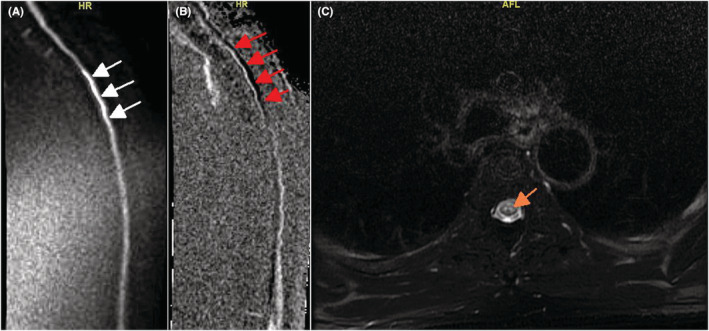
Sagittal plane of MRI spine DWI sequence (A, white arrows) and ADC (B, red arrows) showing restricted diffusion suggesting acute spinal cord infarction from T3 to T5 levels. Axial plane T2‐weighted sequence of MRI spine showing the Owl's Eyes Sign (C, orange arrow)
